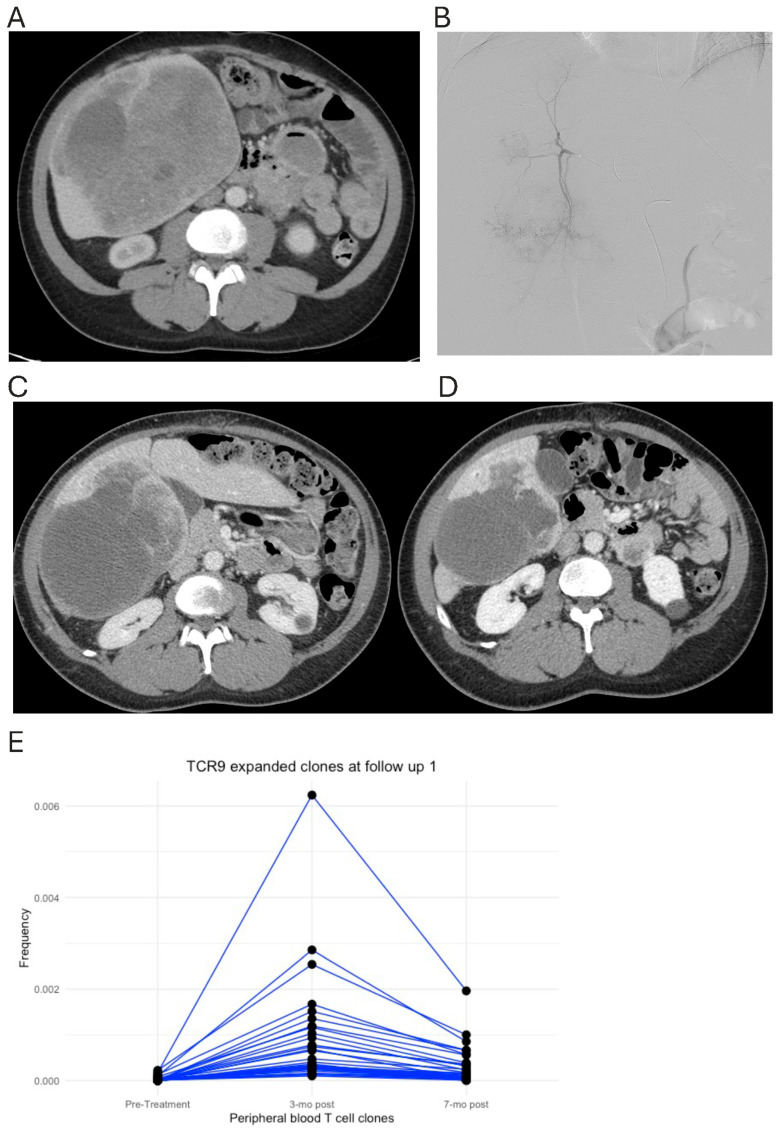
T-cell clonal expansion for a patient with metastatic GIST treated by HAE (TCR9). (A) Baseline CT (B) angiogram during HAE (C,D) follow-up CT 3 months and 7 months after HAE. (E) Frequency tracking of T-cell clonotypes expanded 3 months after HAE. Each line represents a T-cell clonotype.
